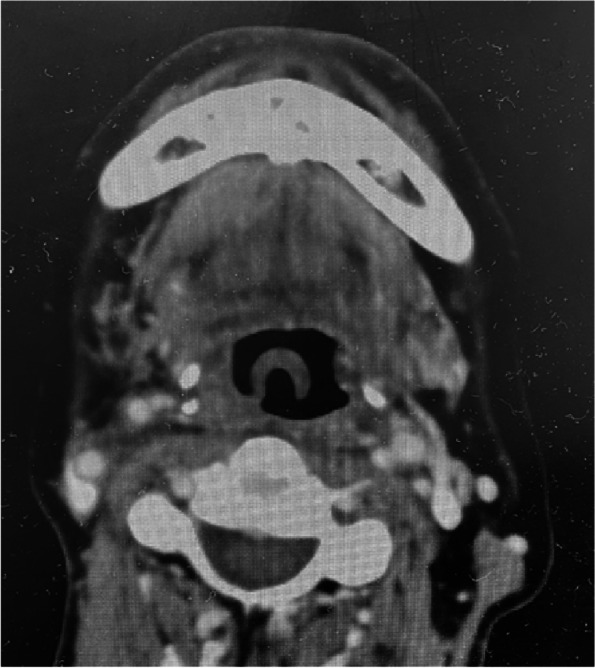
A computed tomography done 2 weeks before surgery. An omega-shaped epiglottis shown in a CT scan
